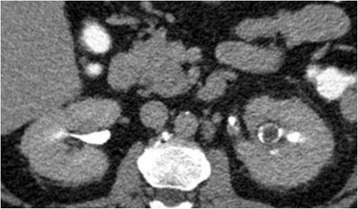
CT scan showed an obstruction of the renal pelvis caused by an amorphous mass (Hounsfield units: 13)
